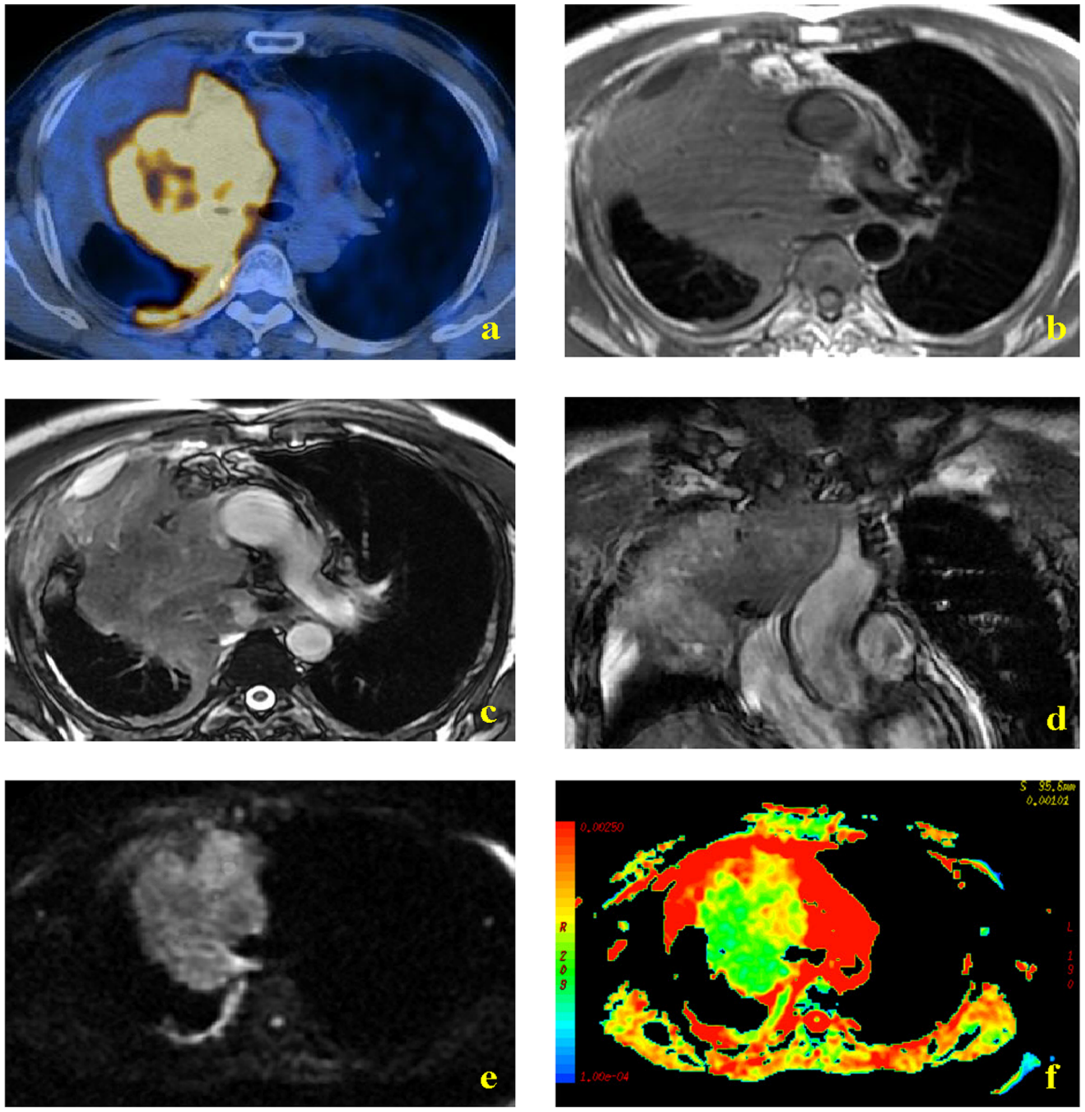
MR and PET/CT images of a 58-year-old man with small cell lung carcinoma. PET-CT indicated greater uptake of FDG by tumor than atelectasis (a). T1W MR imaging showed a soft-tissue shadow in the right upper lobe, but similar signal intensities of the central lung carcinoma and the distal atelectasis were noted (b). FRFSE T2W axial and coronal images indicated hypointensity of the tumor mass relative to the atelectasis (c and d, respectively). DW images obtained with a diffusion gradient of 500 s/mm^2^ allowed easy differentiation of tumor and atelectasis (e and f).
